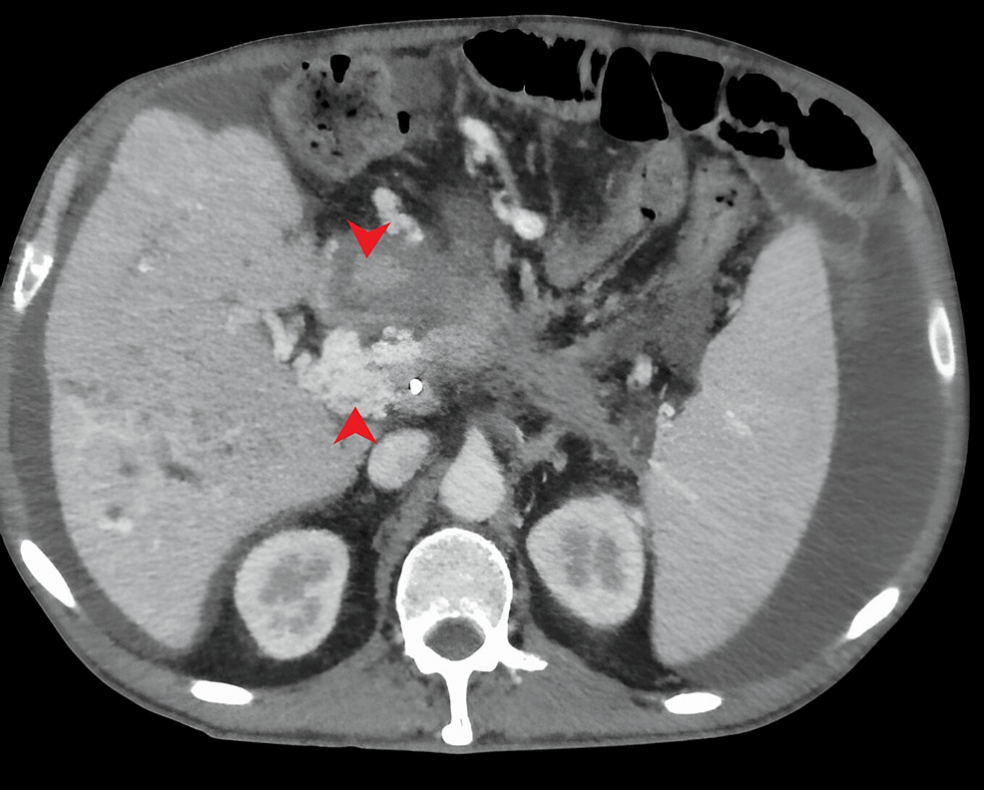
CT scan after 10 months A pancreatic tumor was observed at the level of the intrapancreatic common bile duct, complete destructuring of the pancreas at the level of the head and uncinate process, and multiple hypodense lesions in the left hepatic lobe and upper segments of the right hepatic lobe, in addition to complete thrombosis of the portal and its branches, with significant cavernomatosis/collateral circulation at the level of the hepatic hilum.
